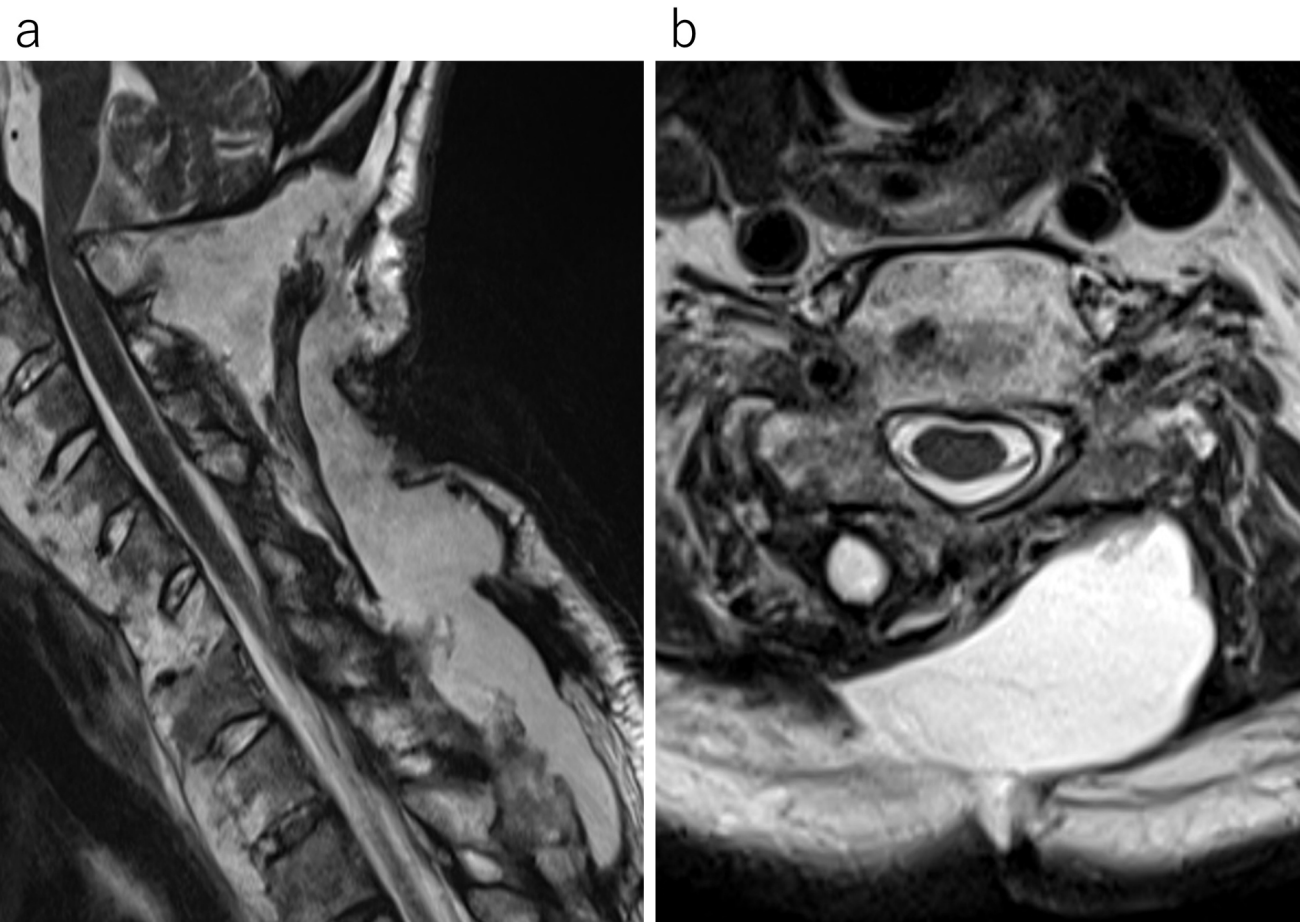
Approximately 1 month postsurgery, subcutaneous swelling was noted, prompting an MRI evaluation. The imaging revealed a pseudomeningocele measuring 14 cm in length on the sagittal plane (a) and 4 cm in width on the horizontal plane (b).
